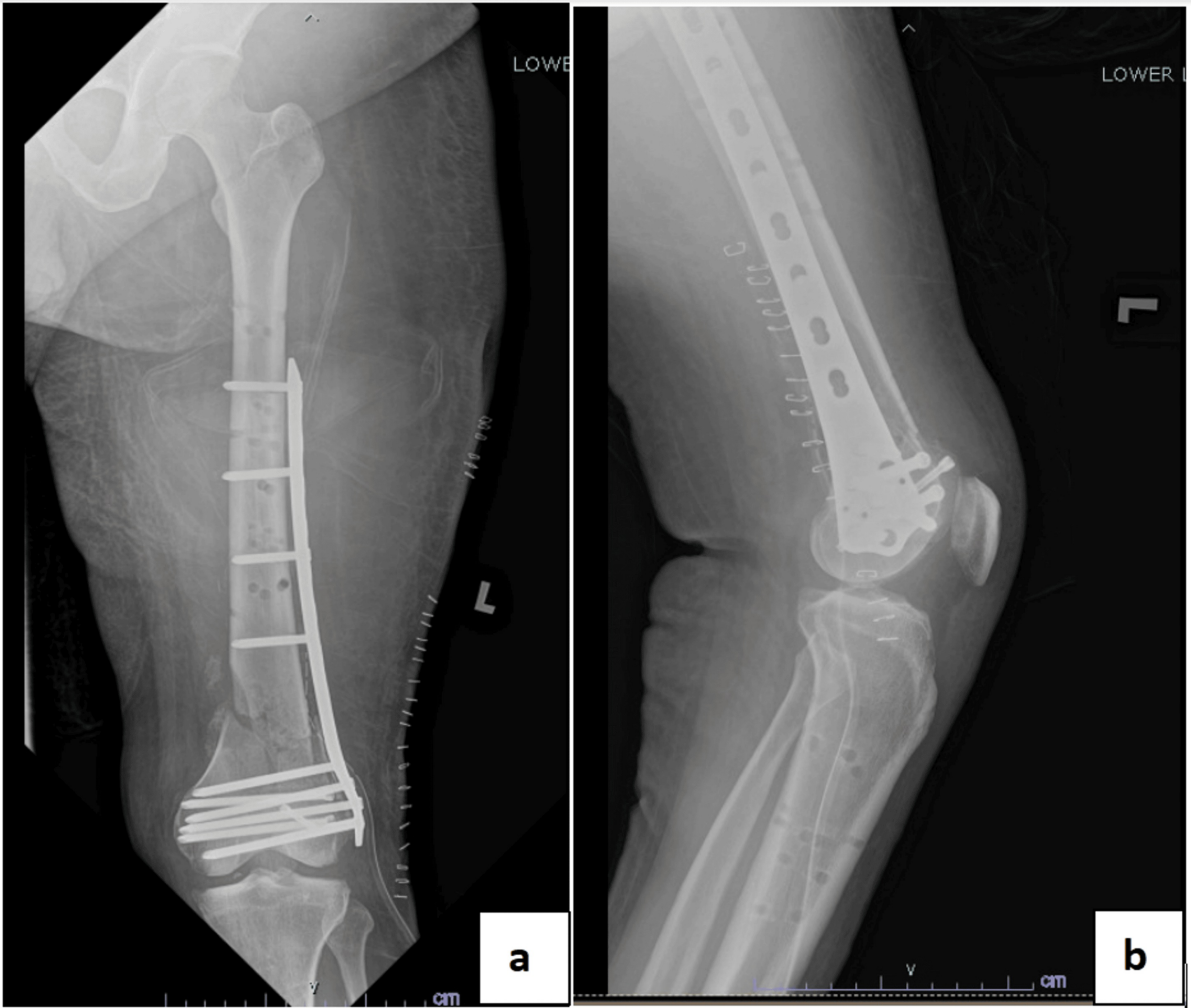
Radiograph of the left femur showing the left distal femur locking plate, performed after the removal of the left cross-knee external fixator. (a) Anteroposterior view. (b) Lateral view.
